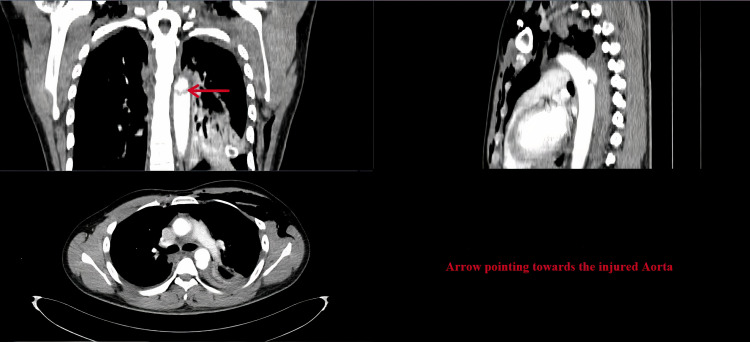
CT angiogram showing injured aorta.
